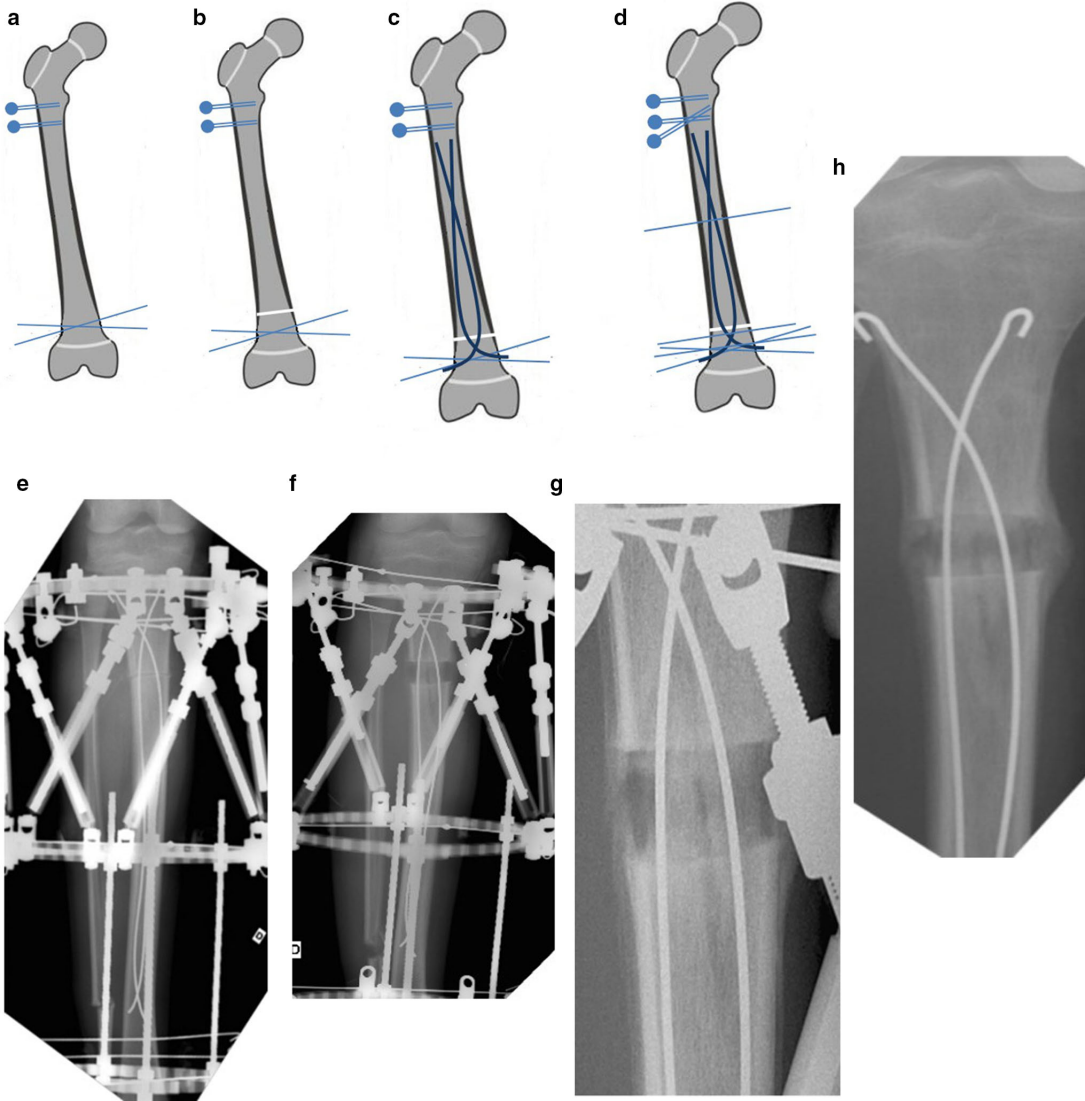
Procedural steps: a application of external fixator with only two proximal half-pins (or wires) and two distal wires; b percutaneous osteotomy; c insertion of intramedullary nails; d external osteosynthesis is completed by adding some wires and half-pins connected to the proximal, medial, and distal rings and arches; e X-ray image of tibia at beginning of lengthening–combined osteosynthesis with FIN and Taylor Spatial Frame^®^; f X-ray image of tibia on day 20 of distraction period; g X-ray image of tibia on day 25 of fixation period, bone consolidation; h X-ray image after frame removal
